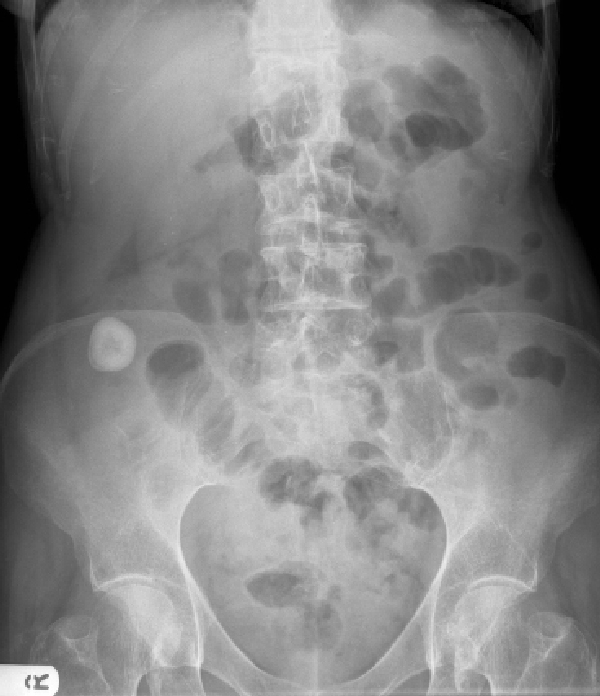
X-ray of the abdomen showing calcification in right iliac fossa.
